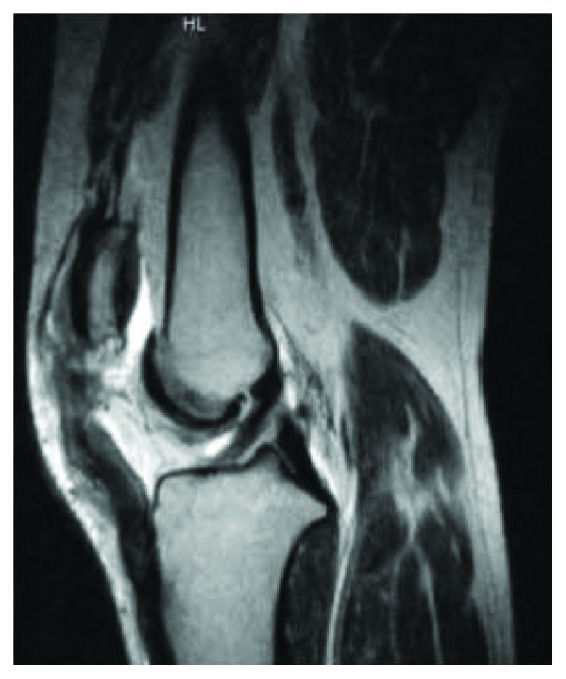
T2-weighted MRI of the right knee demonstrating patella alta and a full thickness tear of his proximal patellar tendon, two months following injury and one month prior to this patient's primary procedure. Insall-Salvati Index = 1.4, Caton-Deschamps Index = 1.34.
